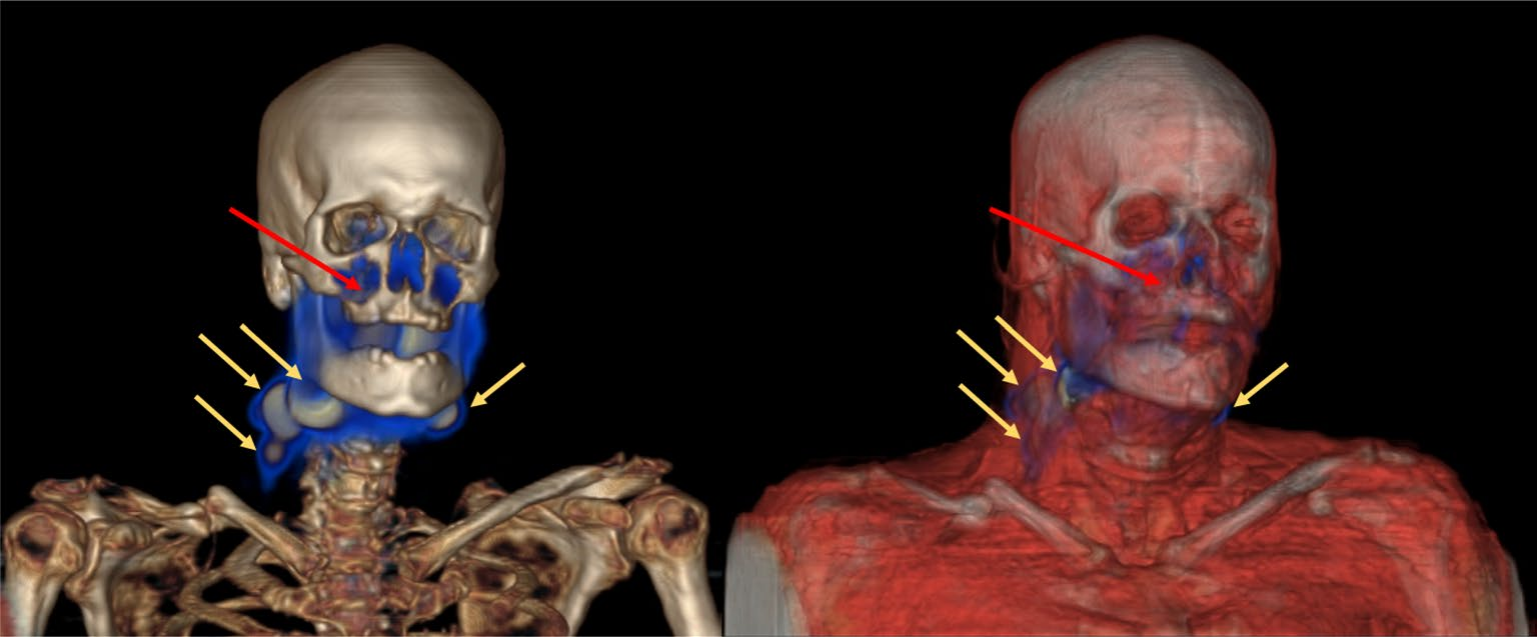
SPECT–CT shows bilateral SNs in a patient with adenocarcinoma in the soft palate, right side. Red arrow = intratumoral injection site; yellow arrows = SNs in the neck.
